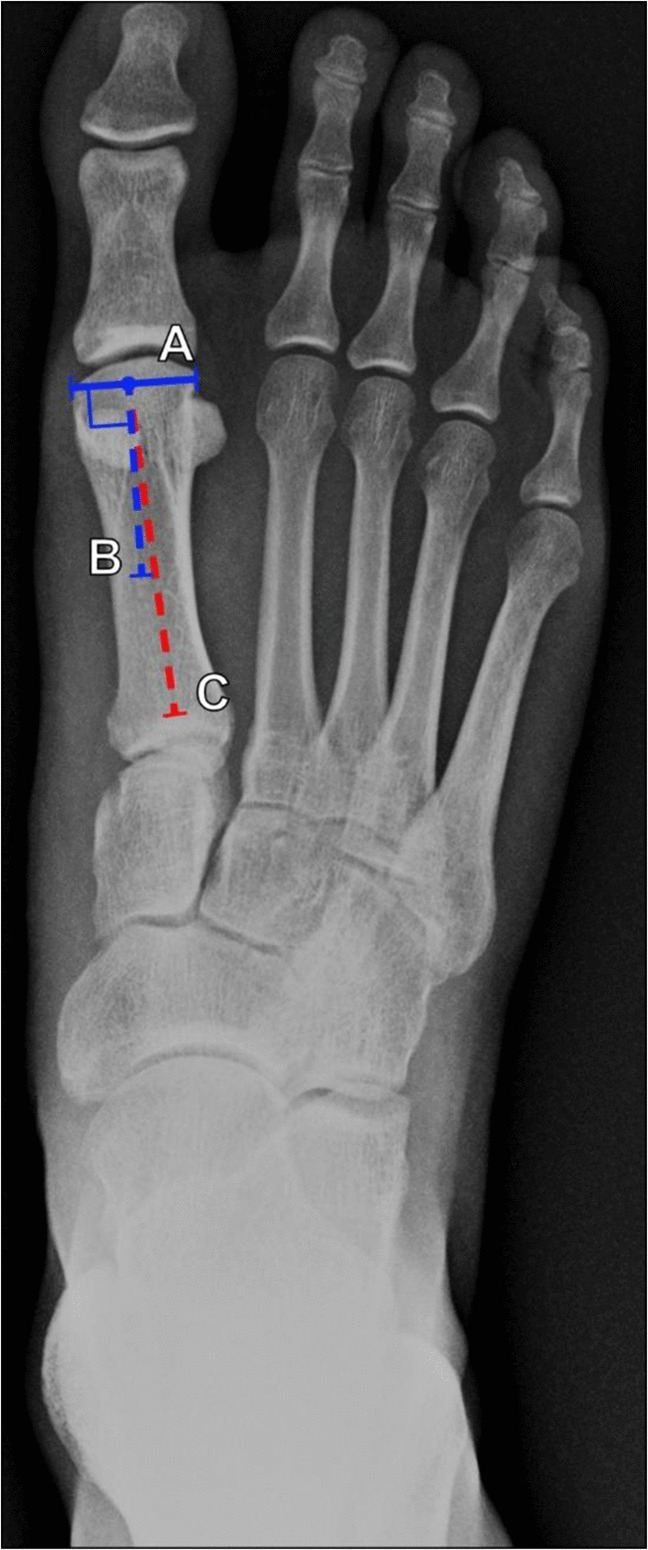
Measurement of the distal metatarsal articular angle (DMAA) on DP radiograph of the foot. Three lines are drawn: a line (A, solid blue line) from the medial to lateral margins of the first metatarsal head articular surface, a line (B, dashed blue line) perpendicular to line A, and a line (C, dashed red line) along the long axis of the first metatarsal. The angle is taken between line B and line C. Generally accepted normal values are ≤ 10°. While a higher DMAA does not necessarily indicate severity of the hallux valgus deformity, it does correlate with hallux valgus progression on longitudinal studies. In some patients, increased metatarsal head pronation may also result in abnormal measured DMAA values
